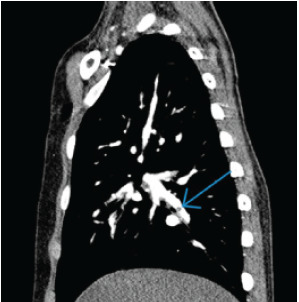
Computed tomography pulmonary angiogram demonstrating a segmental pulmonary embolus (blue arrow).
